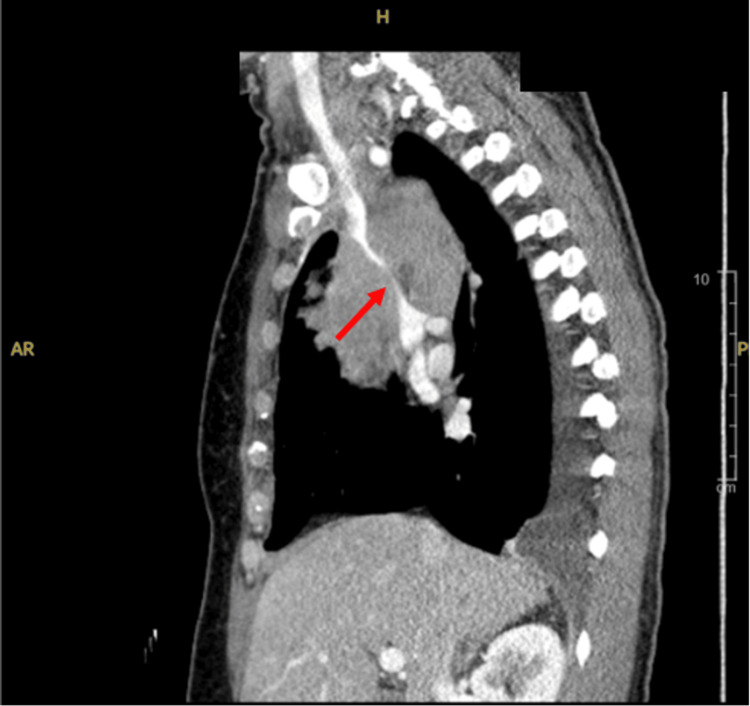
The sagittal computed tomography findings are consistent with superior vena cava (SVC) syndrome, characterized by the near-complete compression of the SVC by a large, invasive anterior mediastinal mass. The SVC appears severely narrowed to a slit-like configuration, and there is an encasement of the left brachiocephalic vein, likely resulting in occlusion.
